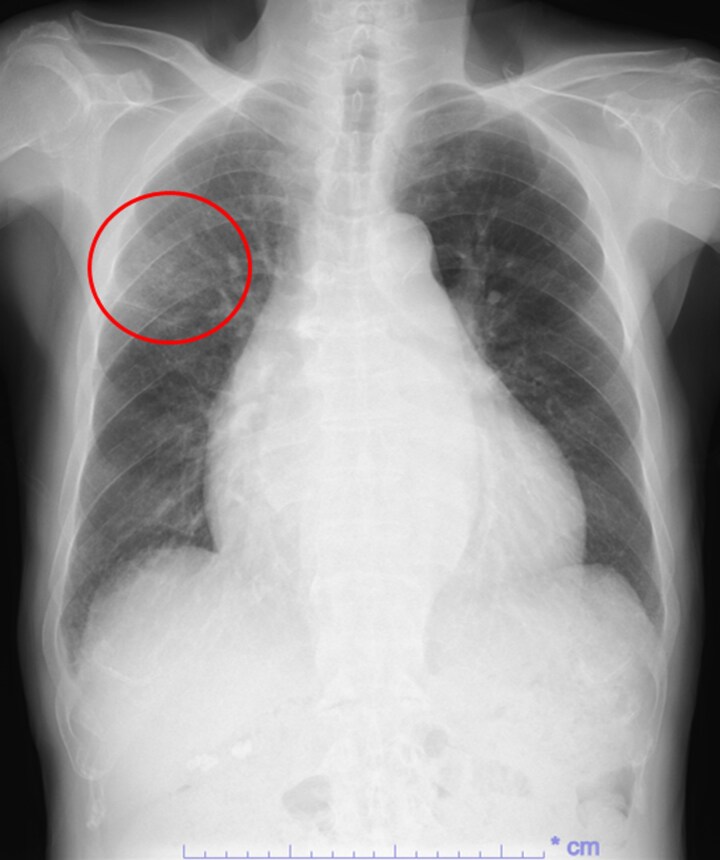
Chest radiograph five days before transcatheter edge-to-edge repair. Chest radiography shows slight residual opacity in the right upper lobe (red circle).
